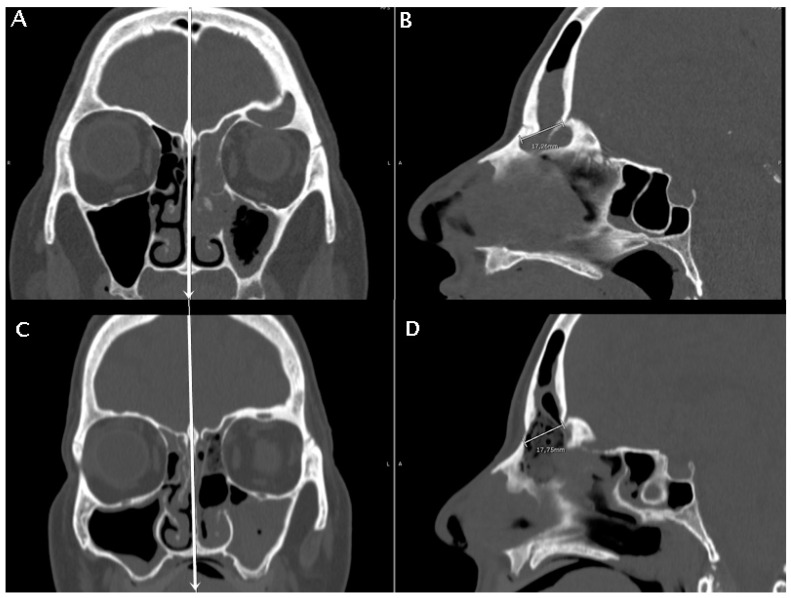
A patient with fronto-ethmoidal inverted papilloma undergoing a DRAF III surgery. The pre-operative CT scan is in (A,B), the post-operative CT scan is in (C,D). Coronal sections are used to identify the mid-sagittal line by the location of nasal septum and crista galli (white vertical lines in (A,C)). The anteroposterior diameter of the frontal sinus ostium is then measured pre- and post-operatively on mid-sagittal sections (B,D). The anteroposterior diameter of the frontal sinus neo-ostium in the post-operatory mid-sagittal images (D) was termed the obtained diameter (OD).
